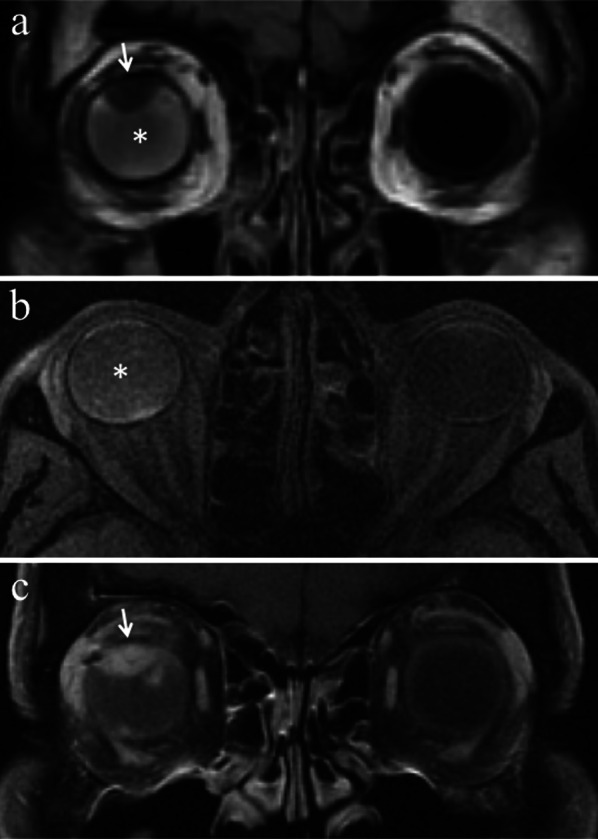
A 37-year-old man with radiation-induced endophthalmitis after proton-beam radiotherapy for choroidal melanoma of the right eye. The patient underwent secondary enucleation three years after radiotherapy because of drug-resistant neovascular glaucoma and painful eye, associated with local recurrence. a Coronal T2-weighted FLAIR (from brain MRI scan) and b axial fat-suppressed T1-weighted images show increased signal intensity of the vitreous body of the right eye (white asterisks) due to vitreous inflammation with protein leakage; note the difference with the physiological water-like signal intensity of the contralateral eye. Along the superior aspect of the right globe, a dome-shaped intraocular mass is detectable, consistent with local recurrence of the choroidal melanoma (white arrow in a). On (c) coronal contrast-enhanced fat-suppressed T1-weighted image, the mass demonstrates enhancement (white arrow)
